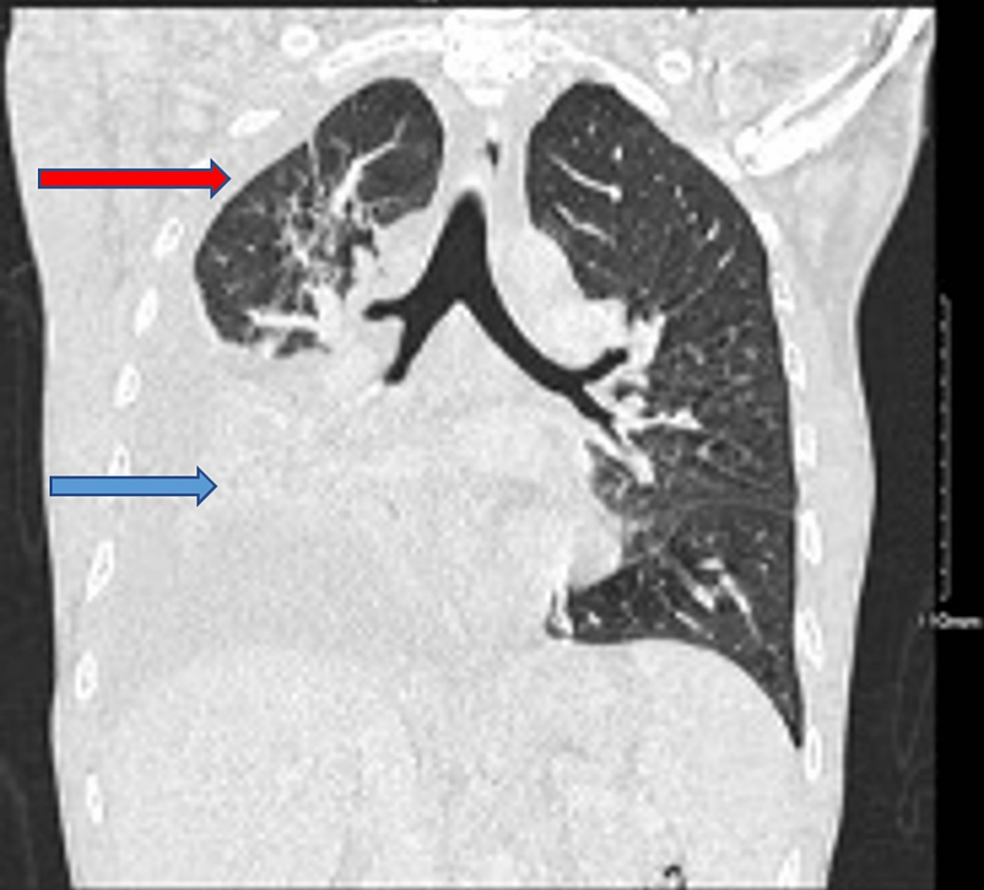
Chest CT shows right-sided consolidation depicted by the blue arrow and thickened pleura shown by the red arrow.
